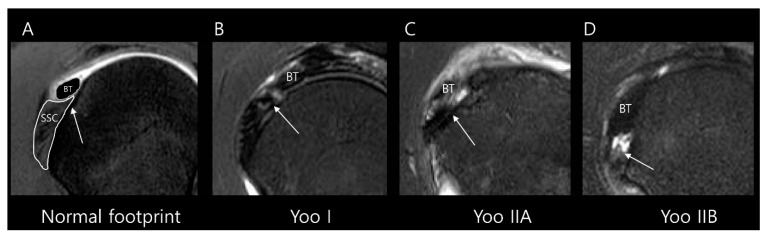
Subclassification of Lafosse type 1 subscapularis tendon (SSC) partial thickness tears according to the Yoo and Rhee classification. On T2-weighted oblique sagittal images with fat suppression, (A) a normal SSC footprint shows a thin tendinous slip (arrow) in the superior portion, which is closely related to the stability of the biceps tendon (BT). Note that most partial thickness tears (Lafosse type 1) originate from this tendinous slip. (B) Yoo type I tear shows fraying or a longitudinal split at the leading edge (arrow). (C) Yoo type IIA tear is defined as the detachment of less than 50% of the first facet (arrow). (D) Yoo type IIB tear is defined as the detachment of more than 50% of the first facet (arrow).
